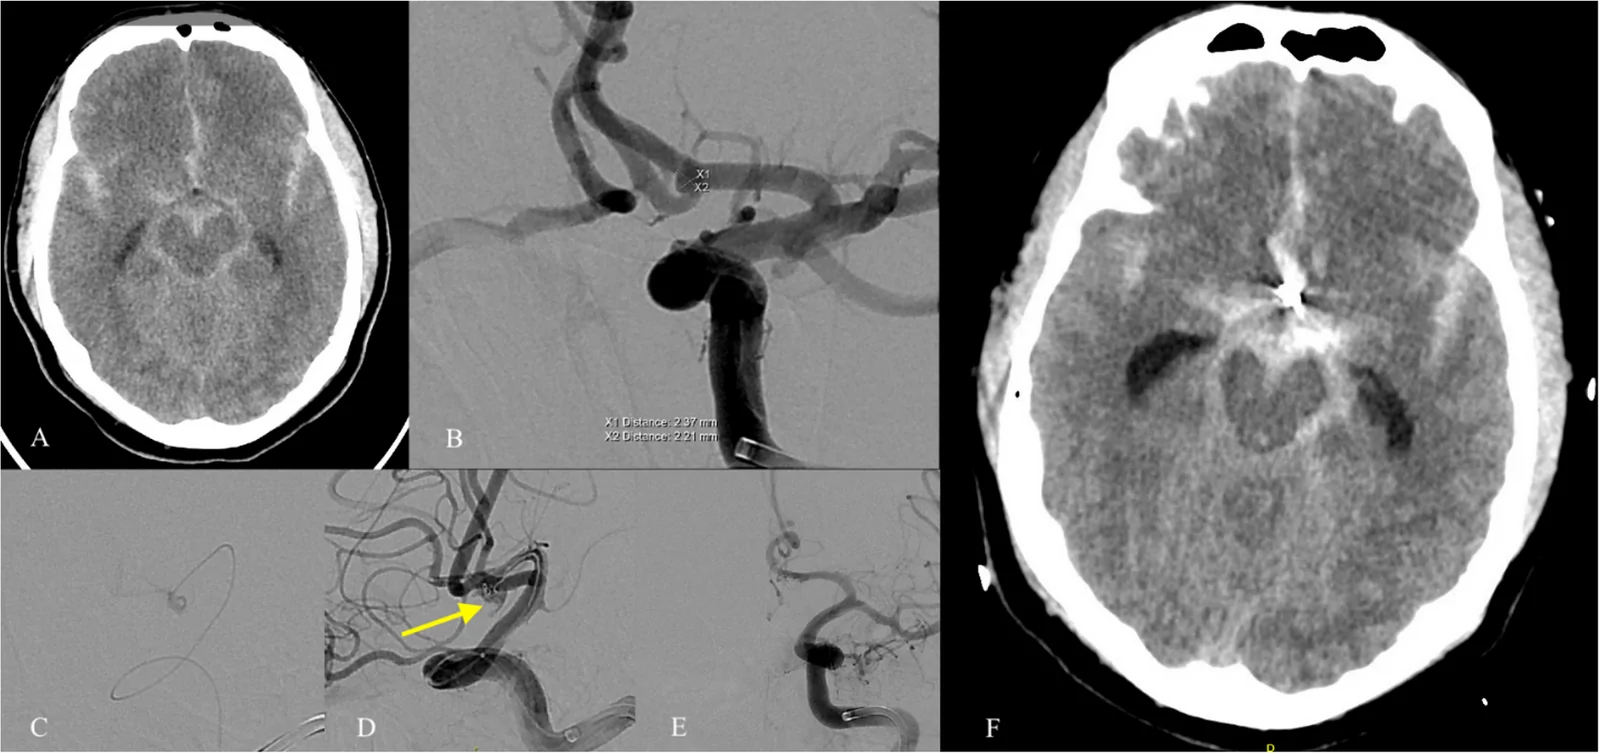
A 25-year-old male smoker presented with acute subarachnoid hemorrhage (SAH). (A) Initial non-contrast head CT demonstrated diffuse acute SAH. (B) Diagnostic cerebral angiography identified a small saccular anterior communicating artery (ACOM) aneurysm, measuring approximately 2.4 × 2.2 mm, with a smooth contour arising from the median ACOM complex. (C) Early intraprocedural fluoroscopy during endovascular access demonstrated stable catheter positioning prior to coil deployment. (D) During coil embolization, angiography demonstrated contrast extravasation consistent with intraprocedural aneurysm re-rupture (Yellow arrow). A balloon was already positioned across the aneurysm neck, allowing for immediate flow arrest and hemostatic control. (E) Subsequent angiographic runs following balloon inflation and continued coil packing demonstrated cessation of contrast extravasation with preservation of the parent vessels. (F) Post-procedural non-contrast CT confirmed interval redistribution and progression of subarachnoid hemorrhage, consistent with the intraprocedural re-rupture event
